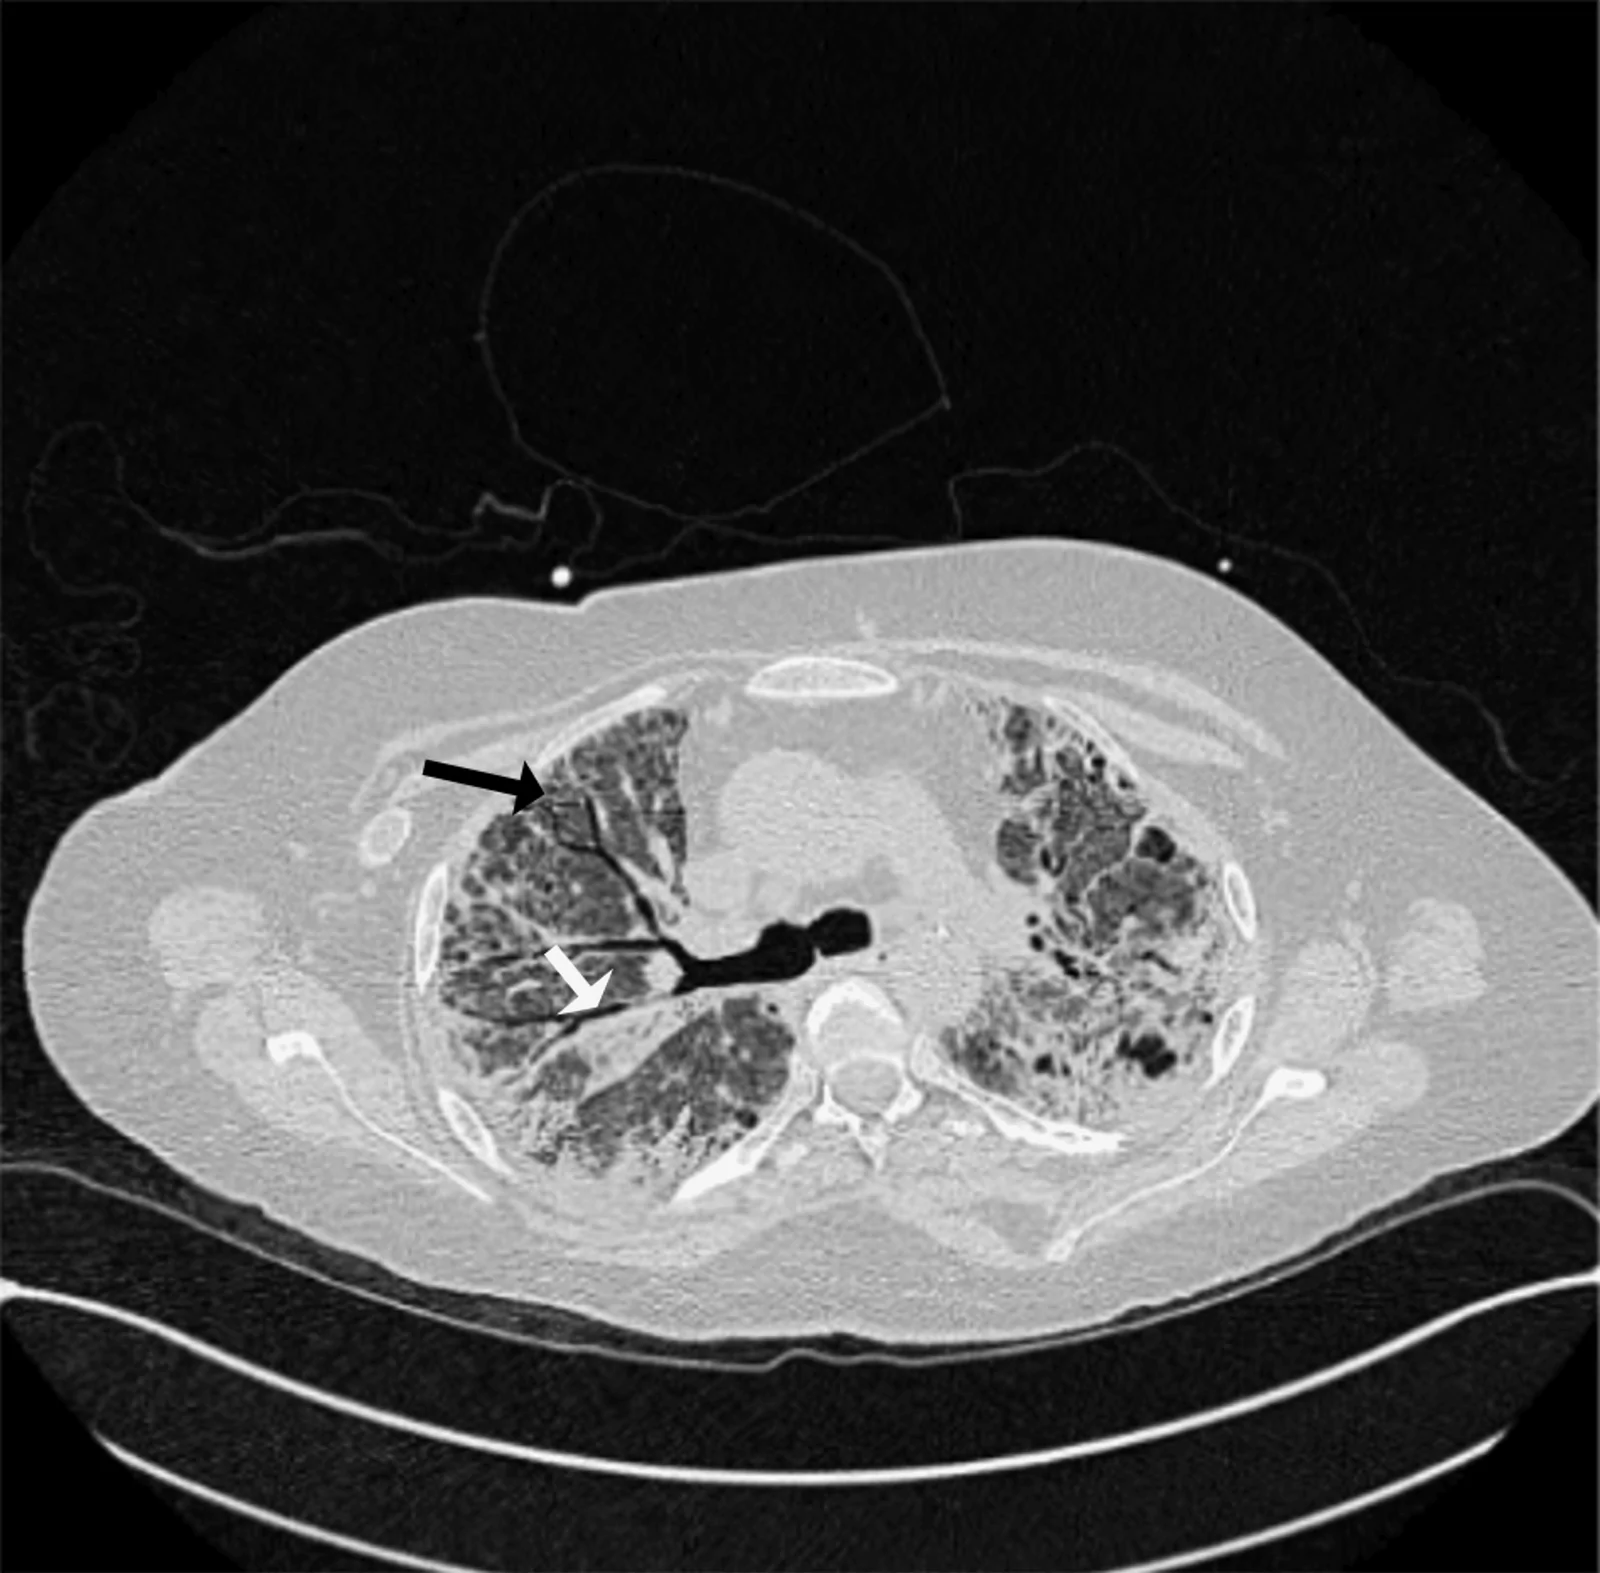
High-resolution chest CT obtained two weeks following initiation of immunosuppression therapy demonstrating fibrotic changes with improvement of previously noticed ground-glass opacities (black arrow) and traction bronchiectasis (white arrow).
